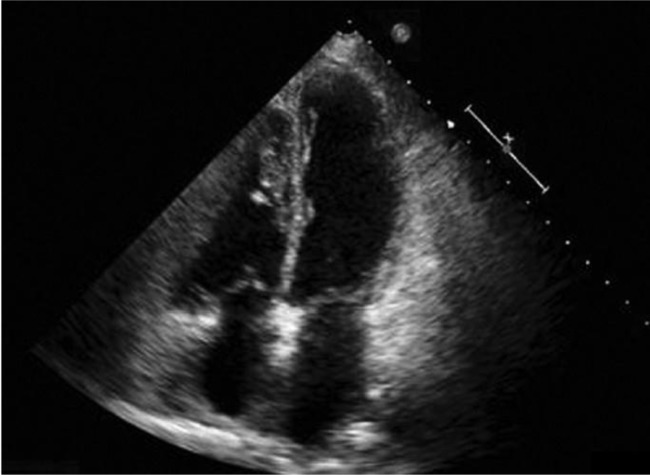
Echocardiography of takotsubo.
